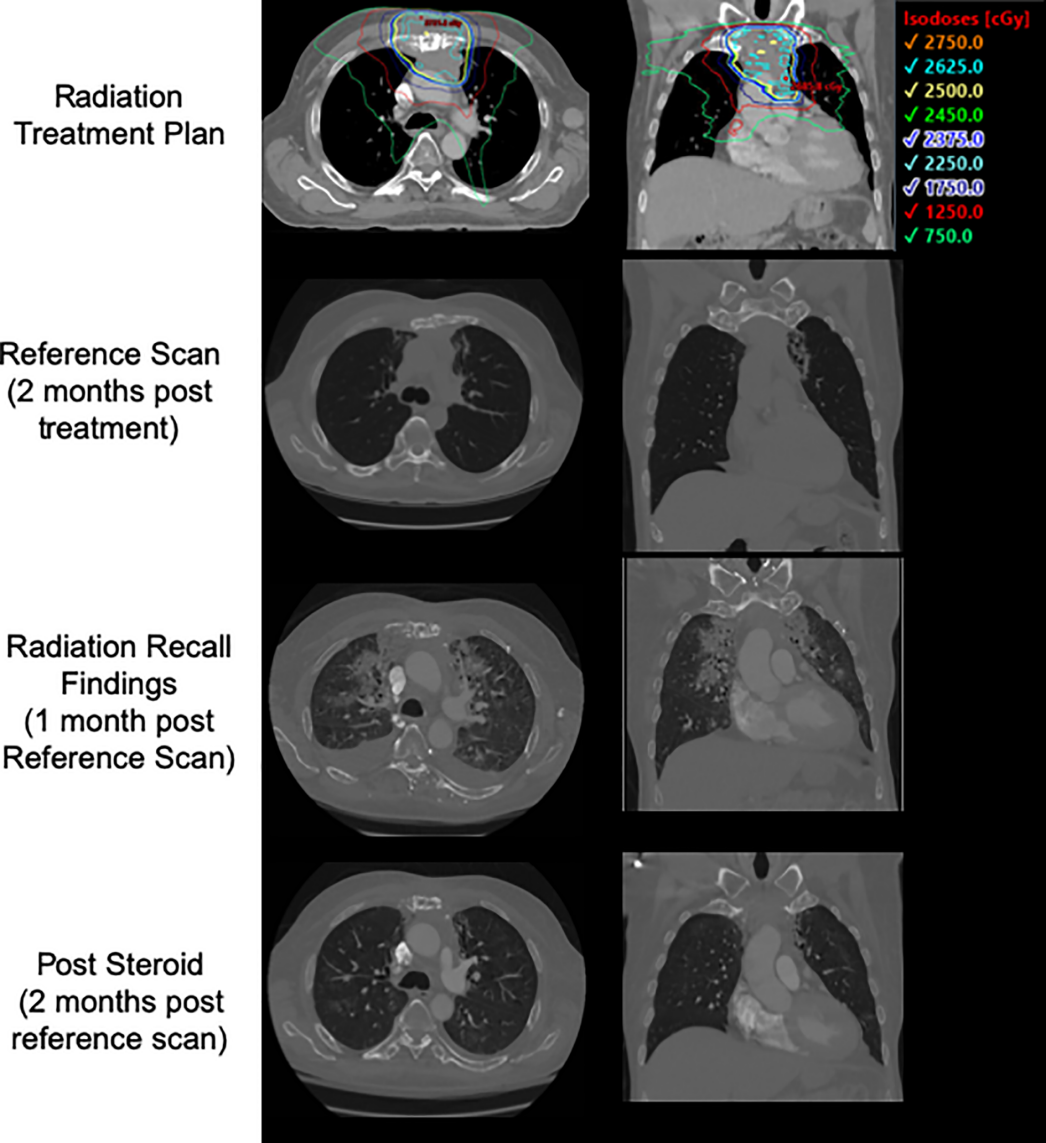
Patient 2's treatment plan, post treatment CT, CT at time of presentation with radiation recall, and CT following course of steroids with interval improvement.
